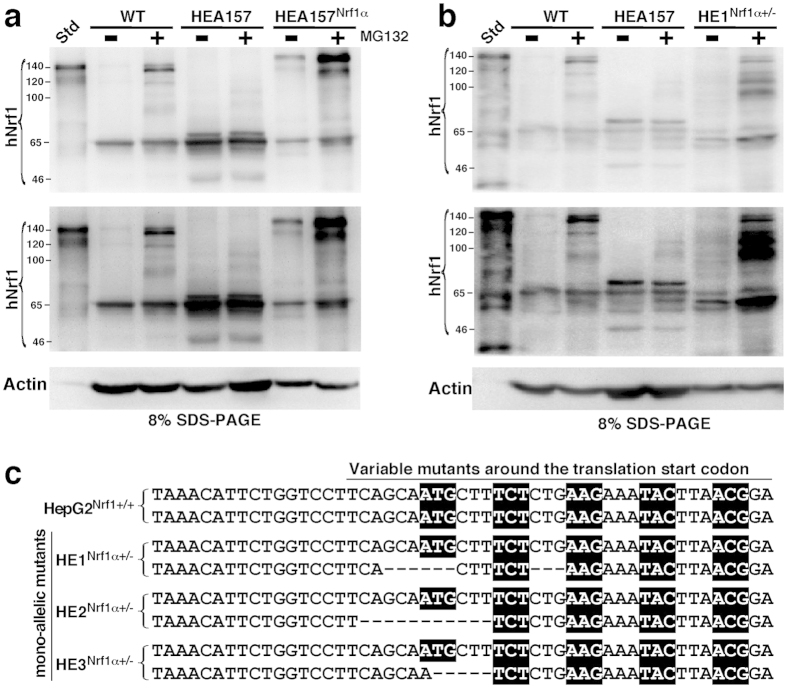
Stable restoration of intact Nrf1α proteins into its knockout cells that are bi- or mono-allelic mutants. (a) A lentivirally-packaged Nrf1α-expressing construct (Lenti-pEZ-Lv203) was transfected, according to the manufacturers’ instructions, into the bi-allelic knockout (*Nrf1α*^−*/*−^) monoclonal cell line (HEA157), and thus the Nrf1α-restored cell line was designated as HEA157^Nrf1α^. Subsequently, western blotting of HEA157^Nrf1α^ and its parent cell lines that had been treated with or without MG132, revealed that stably forced expression of Nrf1α and its derivate proteins was accompanied by a relative decrease of Nrf1β when compared with their expression levels measured in both HEA157 and HepG2 cell lines. The upper two panels show the images obtained from different exposure to X-ray. Of note, other detailed descriptions of HEA157^Nrf1α^ had been not focused herein. (b,c) Three mono-allelic knockout (*Nrf1α*^+*/*−^) monoclonal cell lines (called HE1^Nrf1α+/−^, HE2^Nrf1α+/−^ and HE3^Nrf1α+/−^) had been identified by western blotting (b, and see Fig. S1), target DNA sequencing (c), and other cell biology data (see the legends of Figs S2 to S4). These data are a representative of at least three independent experiments undertaken on separate occasions that were each performed in triplicate. (c) A nucleotide alignment of human wild-type (WT) *Nrf1* and its mono-allelic mutants around the translation start codons that are targeted by TALENs and confirmed by DNA sequencing.
